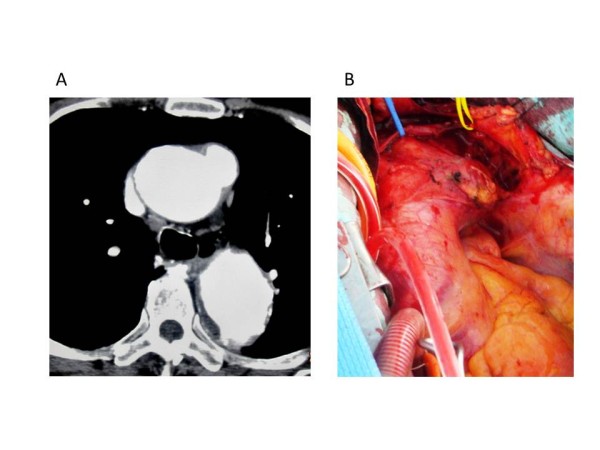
A, B. Preoperative computed tomography scan and intraoperative photograph. The aneurysm was located in the proximal portion of the aortic arch. It was visible opposite the orifice of the brachiocephalic artery.
